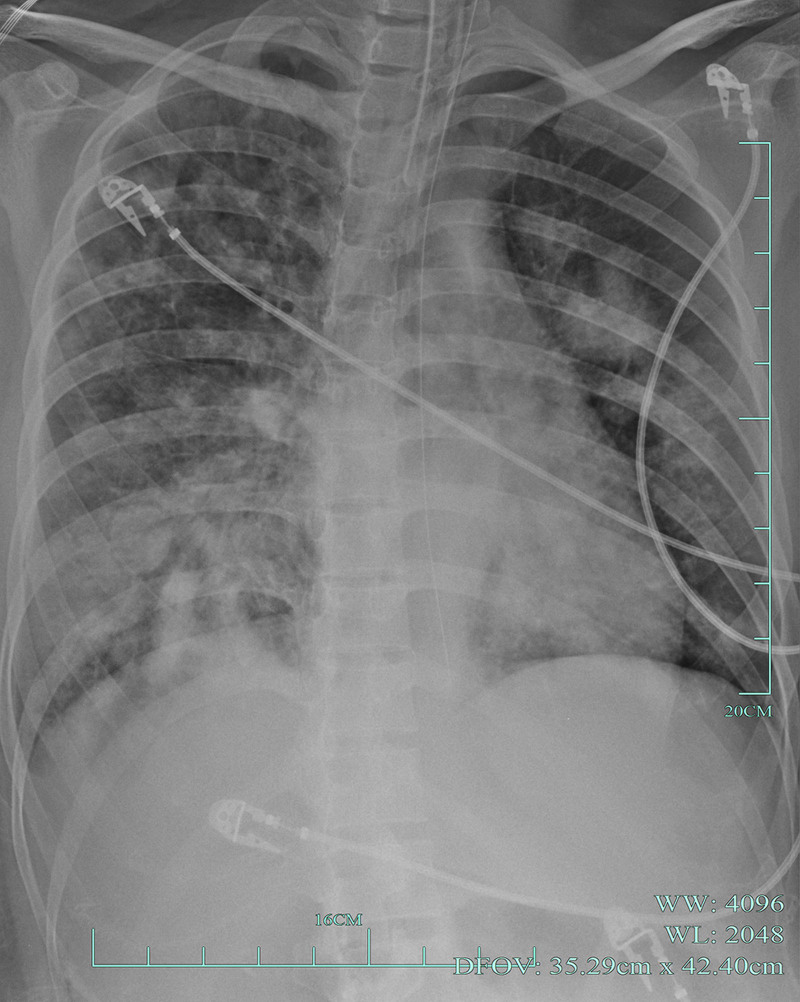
March 4, 2023 chest X-rays (CXRs) showed an increase in multiple infectious lesions in both lungs. CXRs = chest X-rays.
